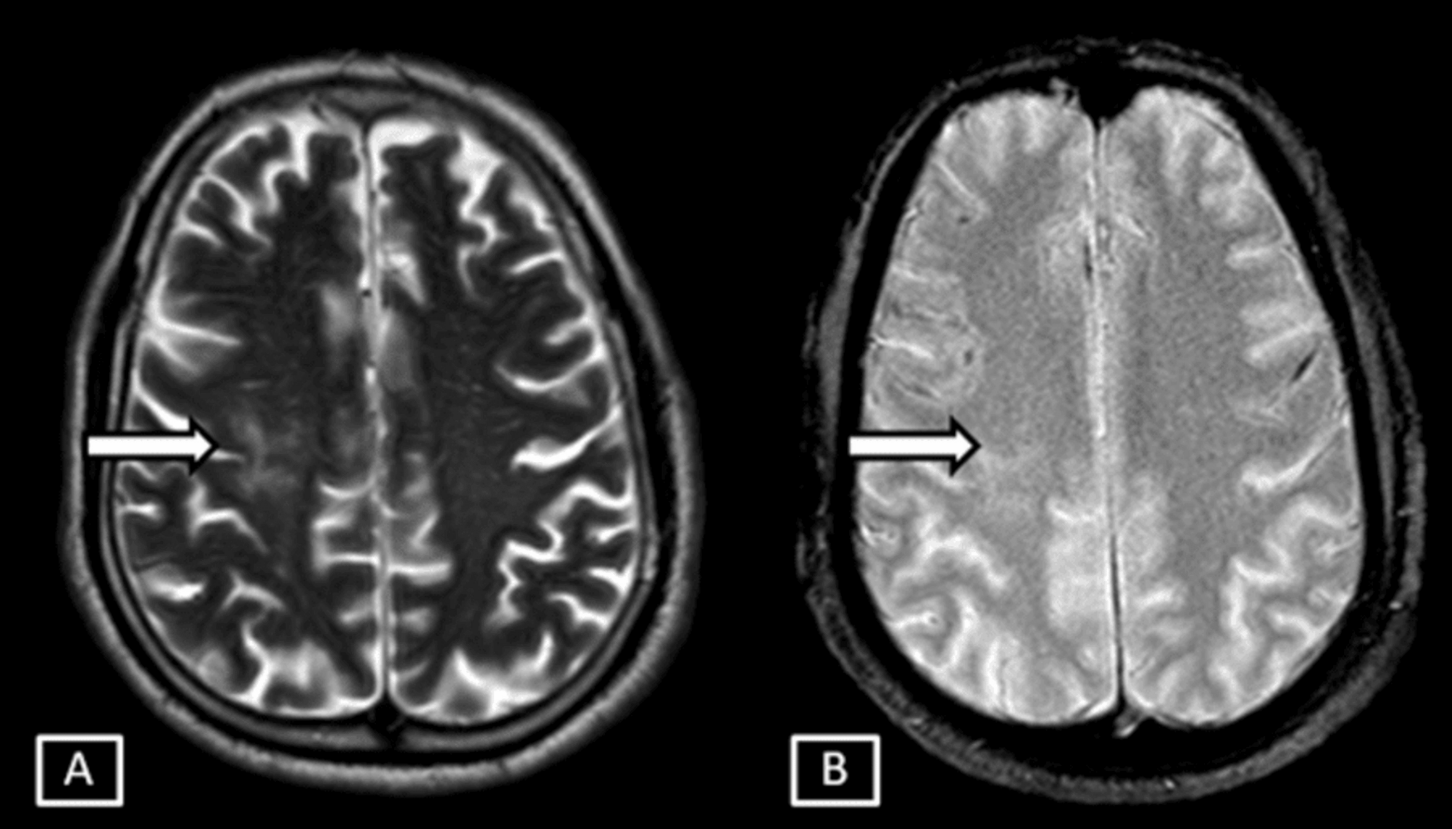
Case 3 MRI brain T2 (A) and GRE (B) sequences. Images show corresponding T2 hyperintense signals with no evidence of any GRE blooming in the area of diffusion restriction suggestive of acute non-hemorrhagic infarct represented by the white arrows. GRE: Gradient Echo
